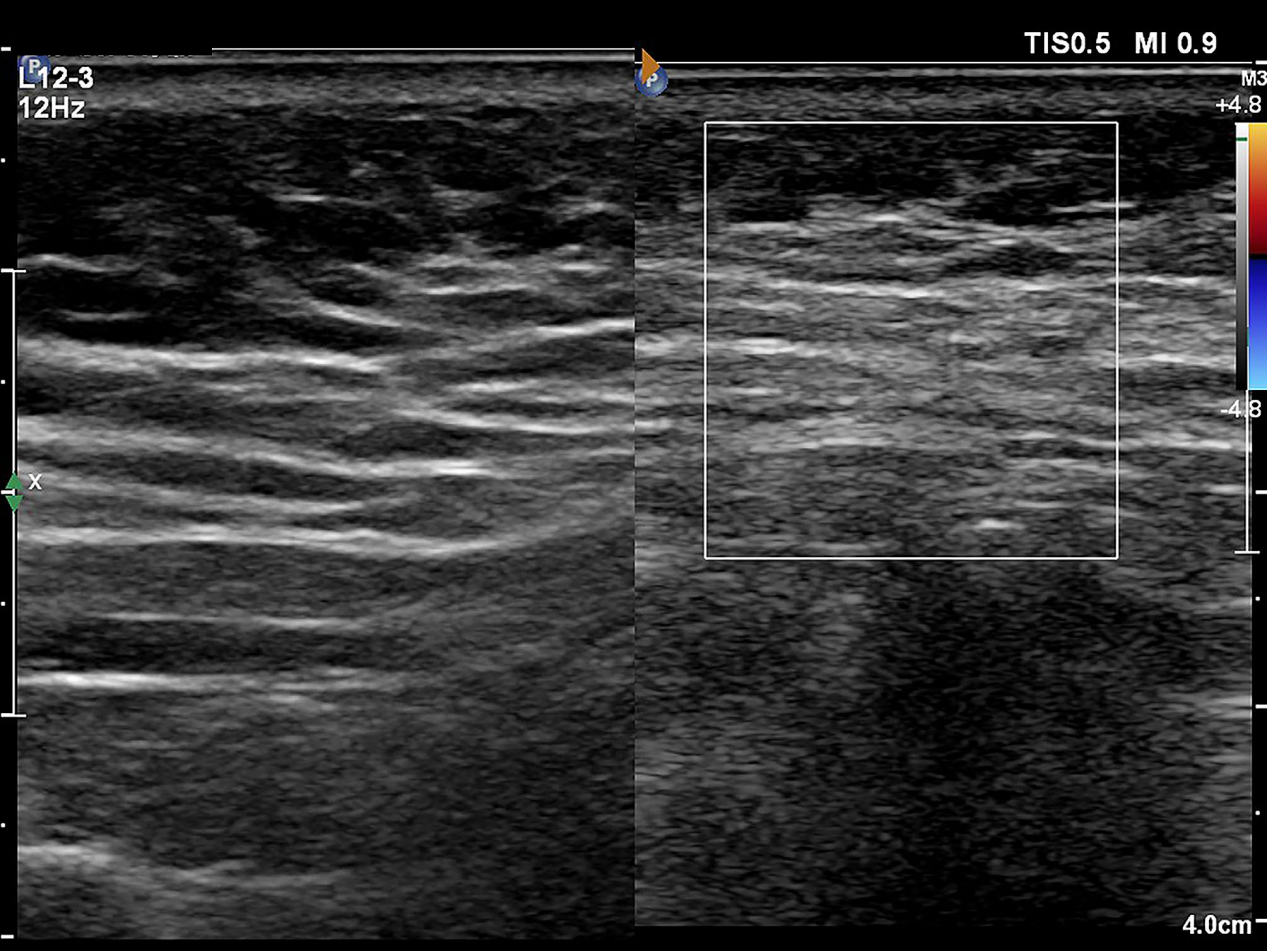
Ultrasonography imaging of the neck mass
